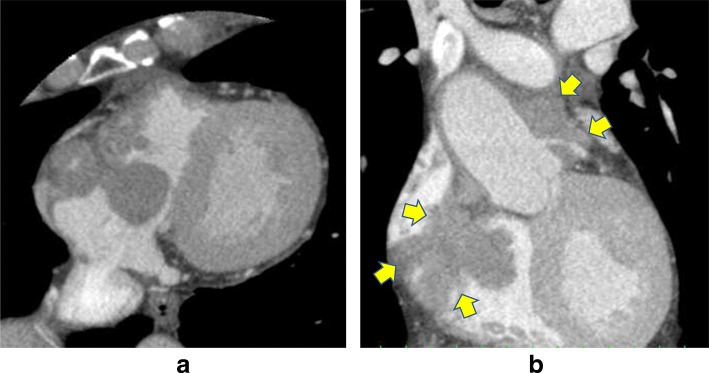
Preoperative contrast-enhanced CT. The big tumor was existed in the right atrium with axial section (a). The right arrows show invasion based on soft tissue intensity near the tricuspid valve above the anterior right ventricle in the region between the aorta and pulmonary artery, and around the pulmonary artery with coronal section (b)
